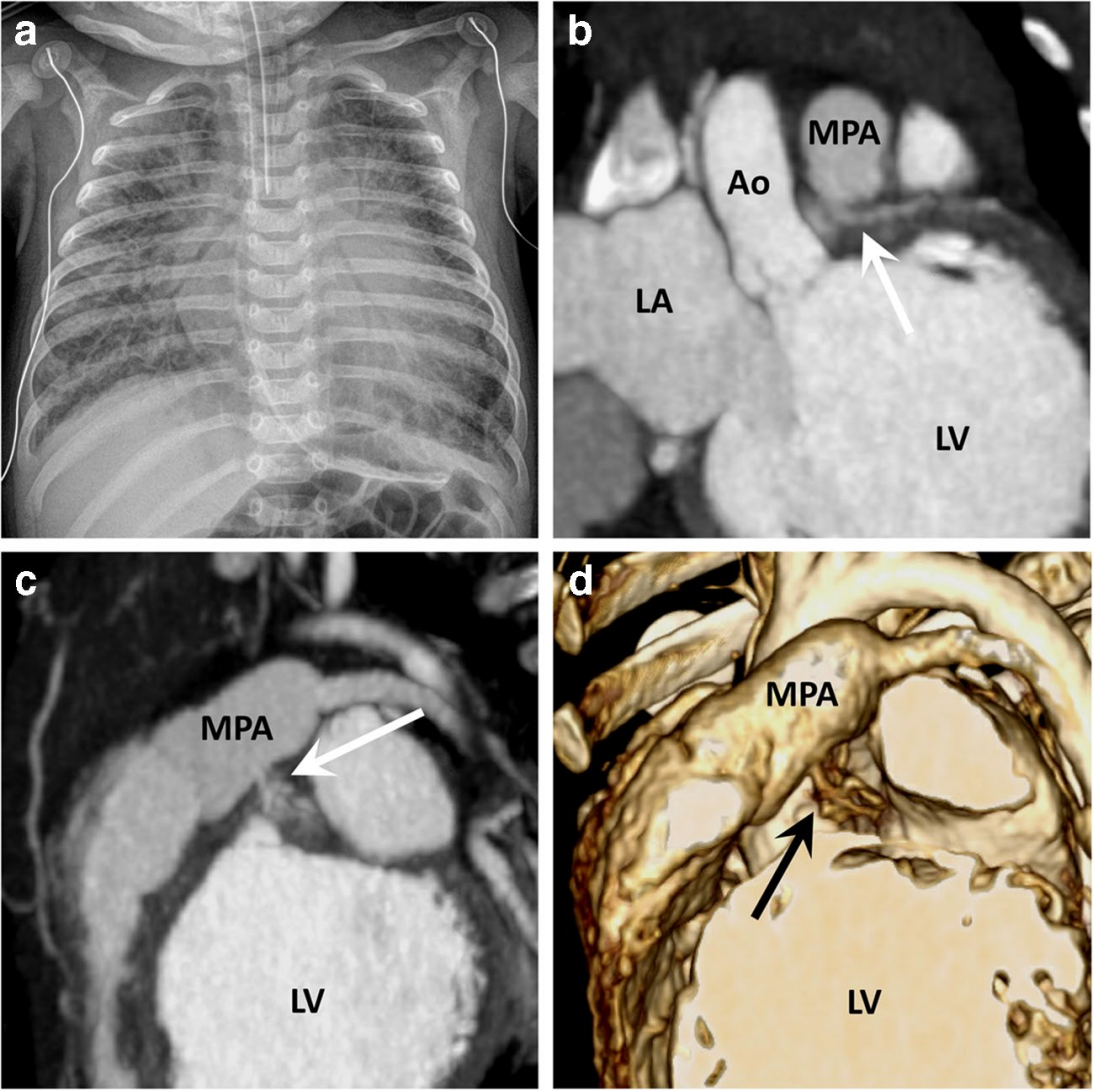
A 9-week-old boy with anomalous origin of the left coronary artery from the pulmonary artery who presented in cardiogenic shock. a Anteroposterior chest radiograph shows moderate to severe cardiomegaly with pulmonary edema. An endotracheal tube is near the carina and a nasogastric tube is in the stomach. b-d Coronal (b) and sagittal (c) contrast-enhanced cardiac computed tomography maximum intensity projection and volume rendering technique reconstructions (d), show the left main coronary artery (LMCA) arising from the undersurface of the main pulmonary artery (MPA) (*arrows*). This patient also has a fine network of collaterals surrounding the LMCA. Note: the dilated left ventricle (LV) due to ischemic cardiomyopathy. *Ao* aorta, *LA* left atrium
